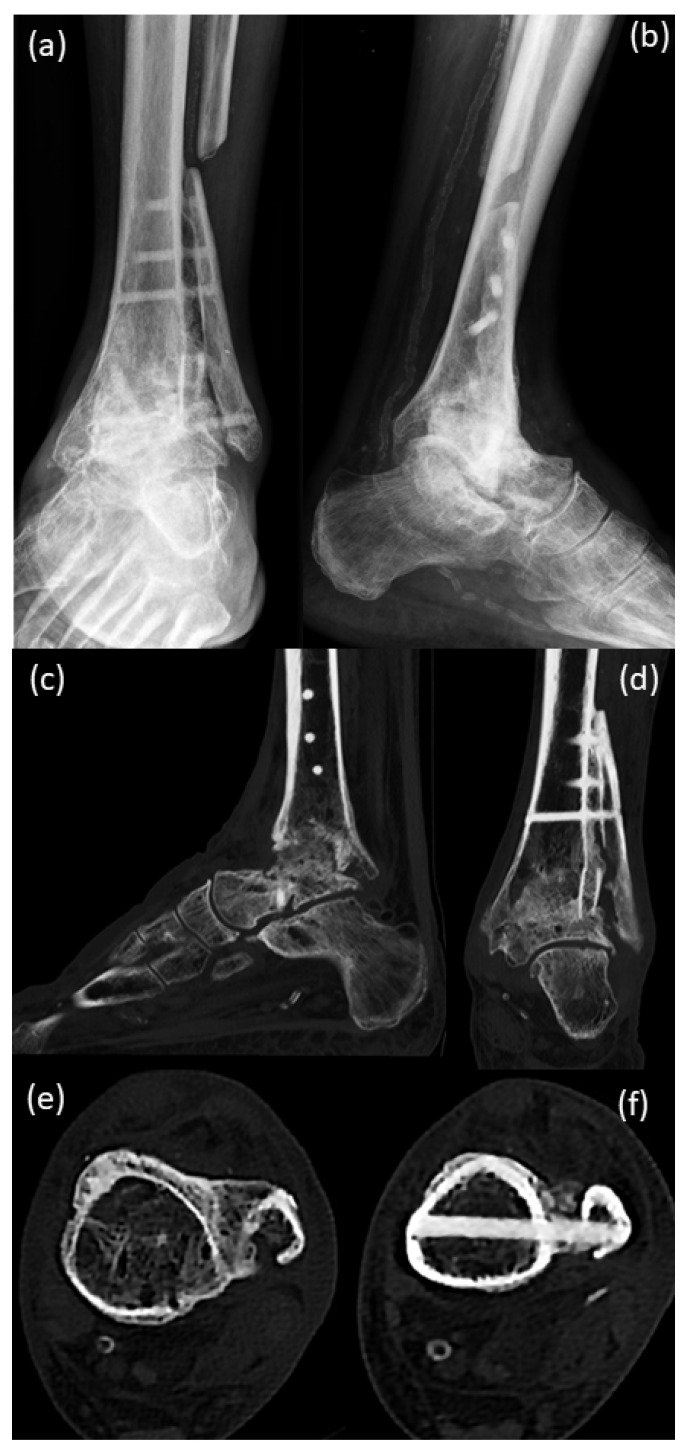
(a,b) X-rays; (c–f) CT scans 1 year after surgery. There is extensive callosal bone superstructing the distal fibular and tibia. It is clearly seen that, in particular, the situation within the distal tibia and the talus is in the status of good bony consolidation. No evidence of bone resorption or insufficiency of screws can be seen.
